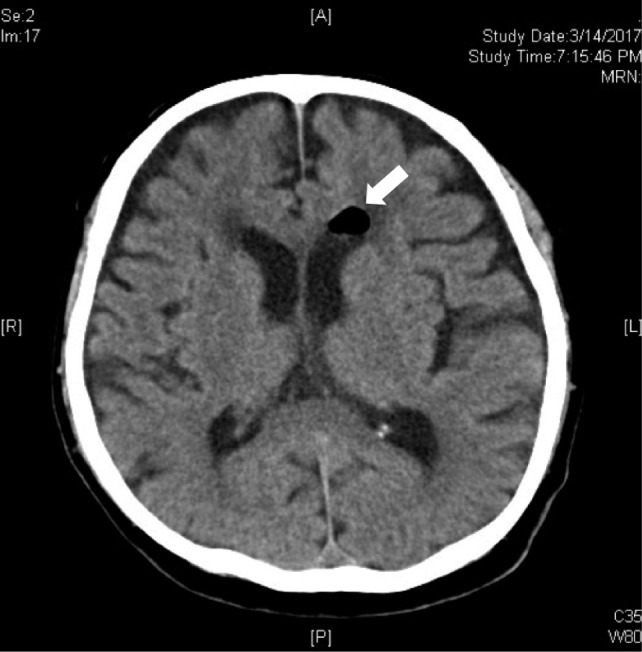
Emergency CT Brain within one hour post-seizure. Focal air pocket in the frontal horn of the left ventricle of the brain noted. (Shown by arrow)
